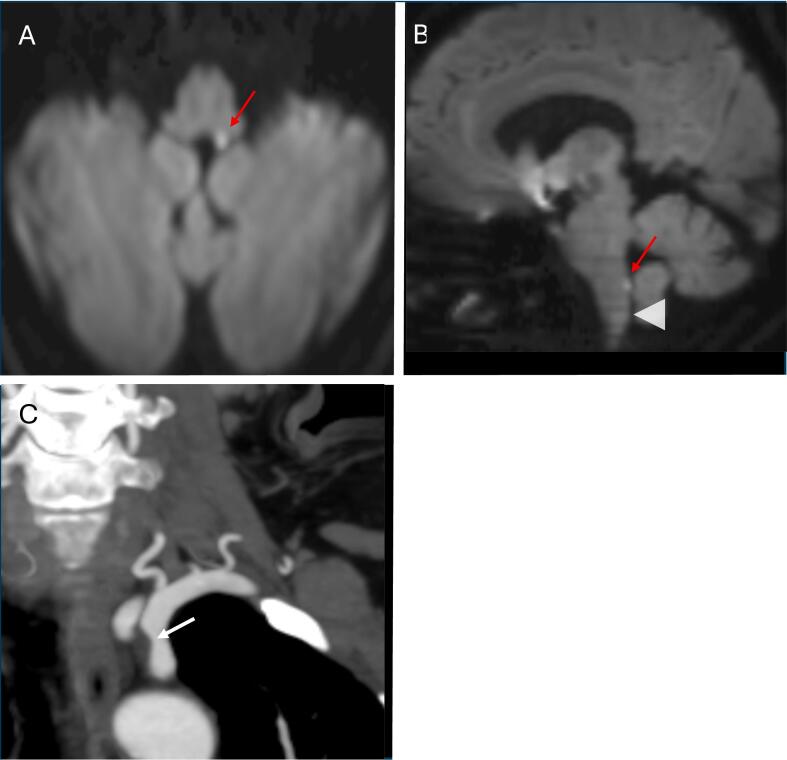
Brain MRI and CT angiography. Axial (A) and sagittal (B) diffusion-weighted imaging show a hyperintensity in the left posteromedial medulla, suggestive of an acute infarction. The topographic relationship between the ischemic lesion (red arrow) and the approximate location of the area postrema (arrowhead) in the dorsal medulla is demonstrated. Coronal (C) CT angiography reveals a non-significant (<50 %) stenosis (white arrow) of the proximal left subclavian artery due to a smooth plaque without calcification. (For interpretation of the references to colour in this figure legend, the reader is referred to the web version of this article.)
